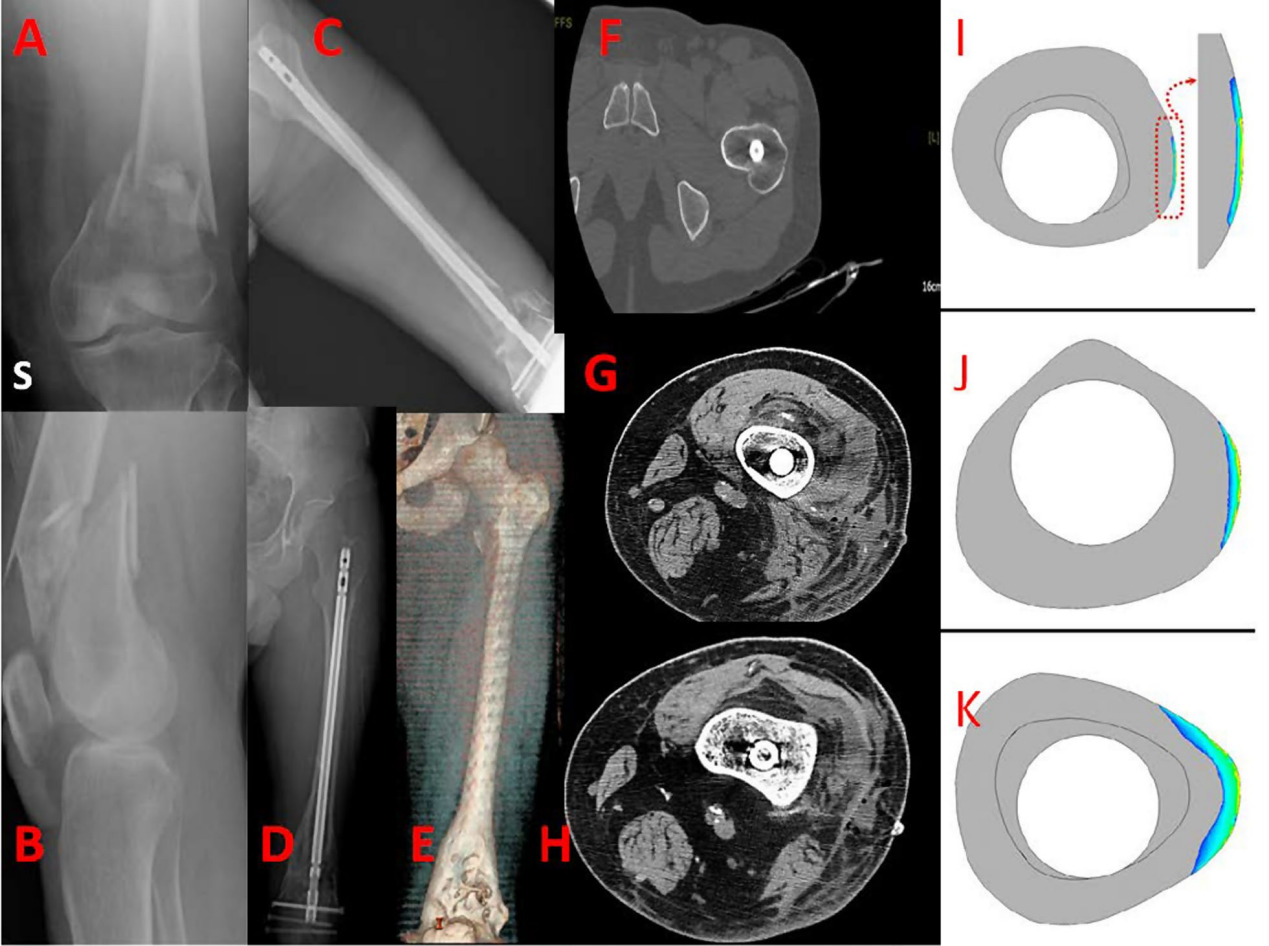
35M, fall from height, 32 C3 type of fracture (A, B). Post-reduction X-ray with fixation with non-locking retrograde nail (C). X-ray and computed tomography (CT) scan at 12 months post-operation show satisfactory fracture healing (D, E). Absence of stress shielding of the nail is shown in F–H comparing images with biomechanics representations (I–K) proposed by Shih et al.
